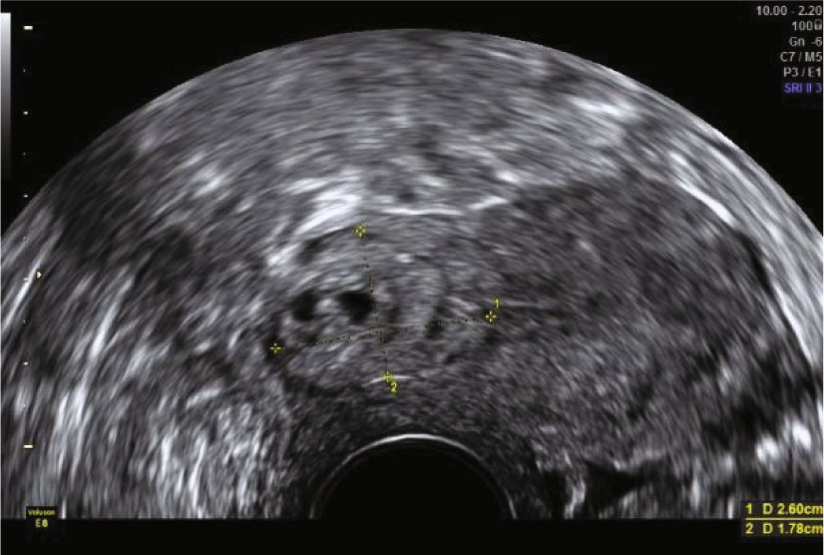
Transvaginal ultrasonographic study of the pelvic-abdominal region showing a retroverted uterus, with a biometry of 64 mm x 38 mm. The uterine morphology was regular, and the myometrial ultrasound pattern was homogeneous. Ultrasound signs of cavitary pathology were not observed. The endometrial thickness was 2 mm. At the level of the cesarean section scar, a 29x16 mm nodular image was observed, with a heterogeneous pattern.
